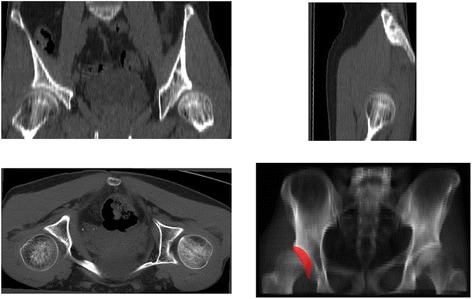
Example of severe dysplasia. Patient 3 exhibited severe dysplasia, as visible in the CT reformats and surface view. In the digitally-reconstructed AP radiograph, the acetabulum is highlighted
